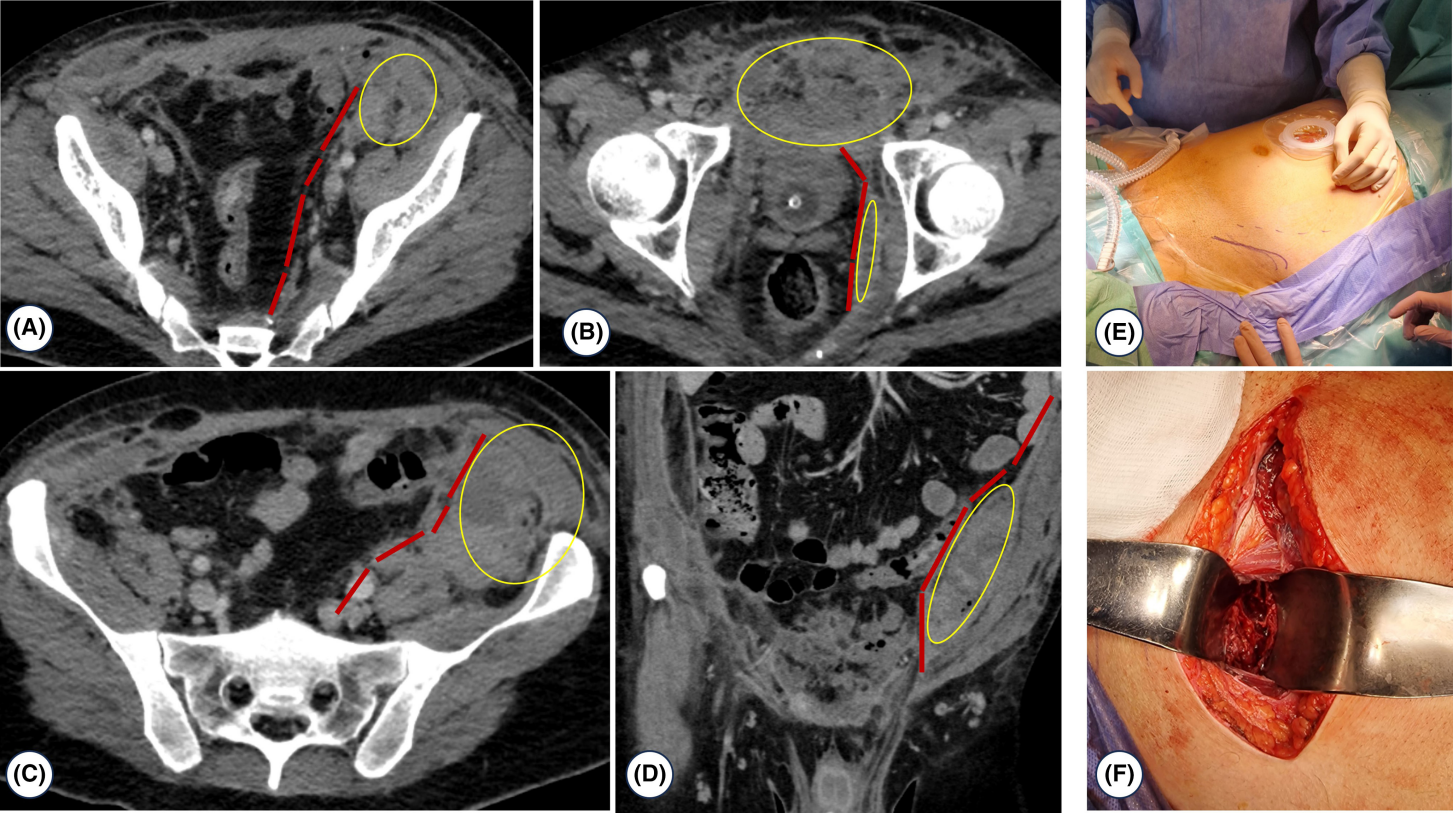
CT scan 4 weeks later (A, B, C, and D): Fluid‐air collections in the abdominal wall, space of Retzius, perirectal area with pelvic extension, and a new collection near the left iliac fossa. Fournier's gangrene resulted in air dissection throughout the perineal fasciae, extending to the fasciae of the abdominal wall muscles (necrotizing fasciitis spread through fascial planes—spread cranially through the space of Retzius). The infected extraperitoneal collection in the left iliac fossa was attributed to a spontaneous infected hematoma caused by therapeutic anticoagulation; an extraperitoneal surgical drainage of this infected organized hematoma was performed (E, F); red dashed lines correspond to the peritoneum; yellow circles correspond to the fluid‐air collections.
